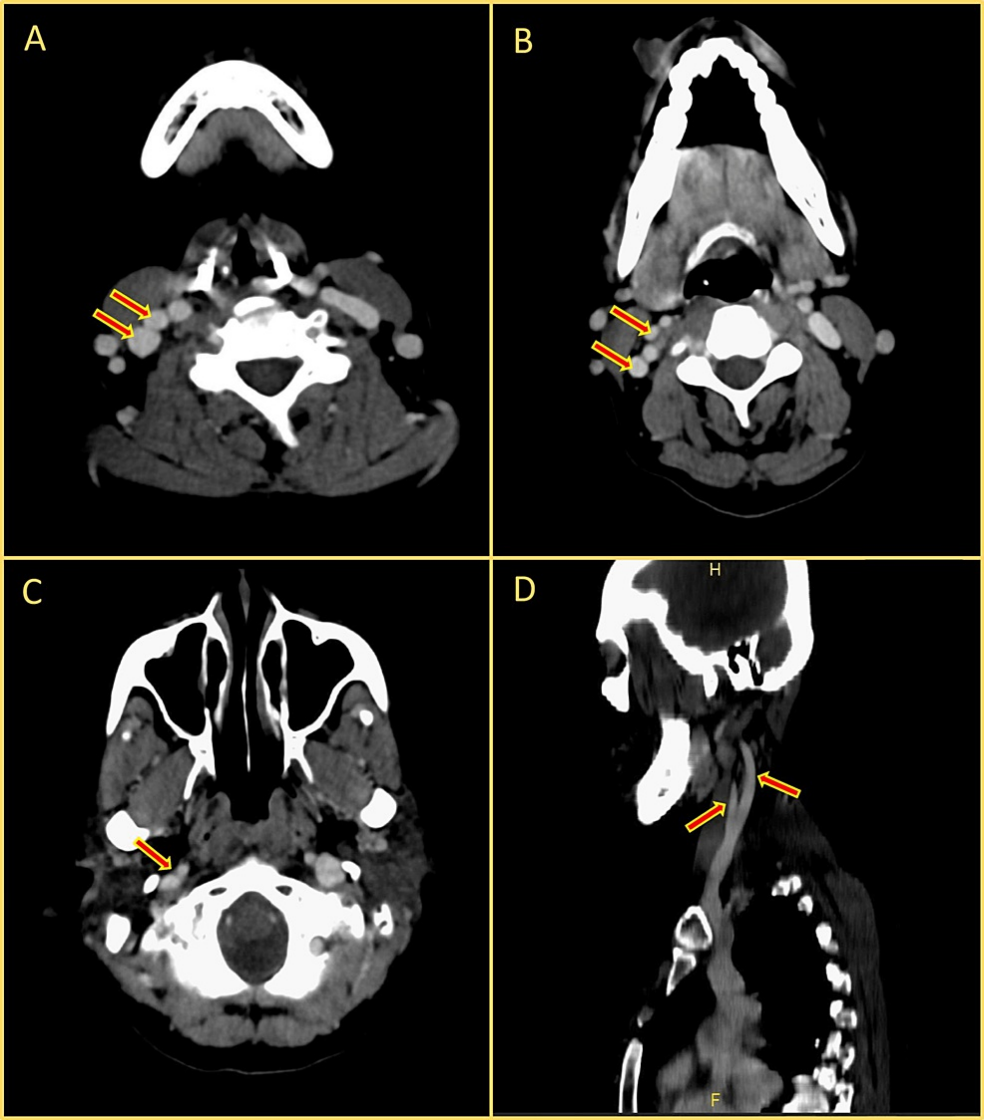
Preoperative contrast-enhanced computed tomography images showing the fenestration in axial and sagittal views A - Double IJV noted in axial view, B - Fenestration of IJV noted at the level of hyoid in axial view, C - Fusion of fenestrated IJV noted near exiting from jugular foramen in axial view, D- IJV fenestration in sagittal view. IJV: internal jugular vein
